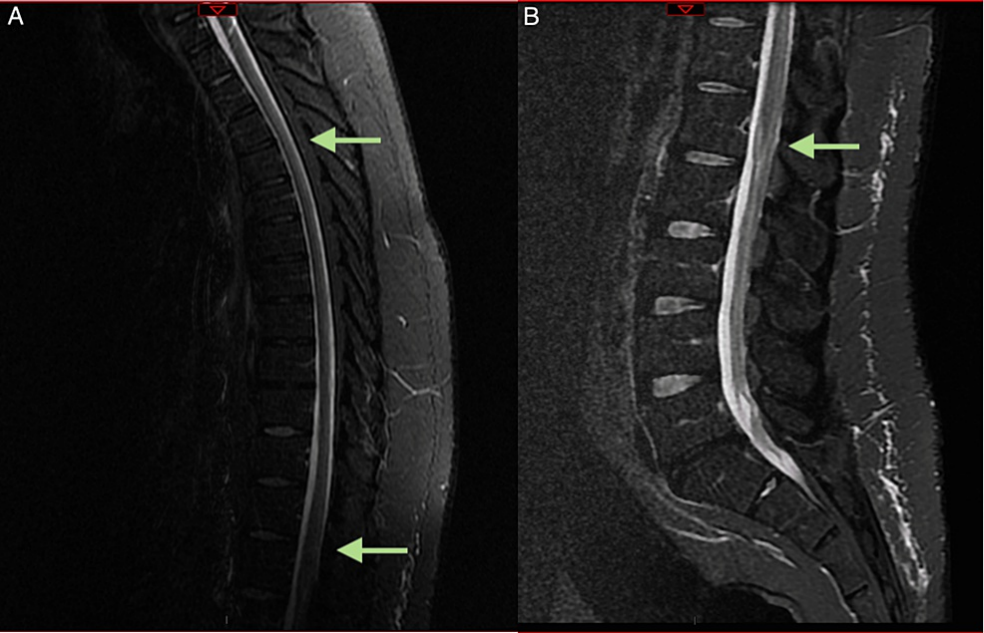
(A) Dorsal and (B) lumbar spine MRI images six months after treatment, showing imagiological improvement in sagittal short-tau inversion recovery sequence (green arrows) MRI: magnetic resonance imaging
